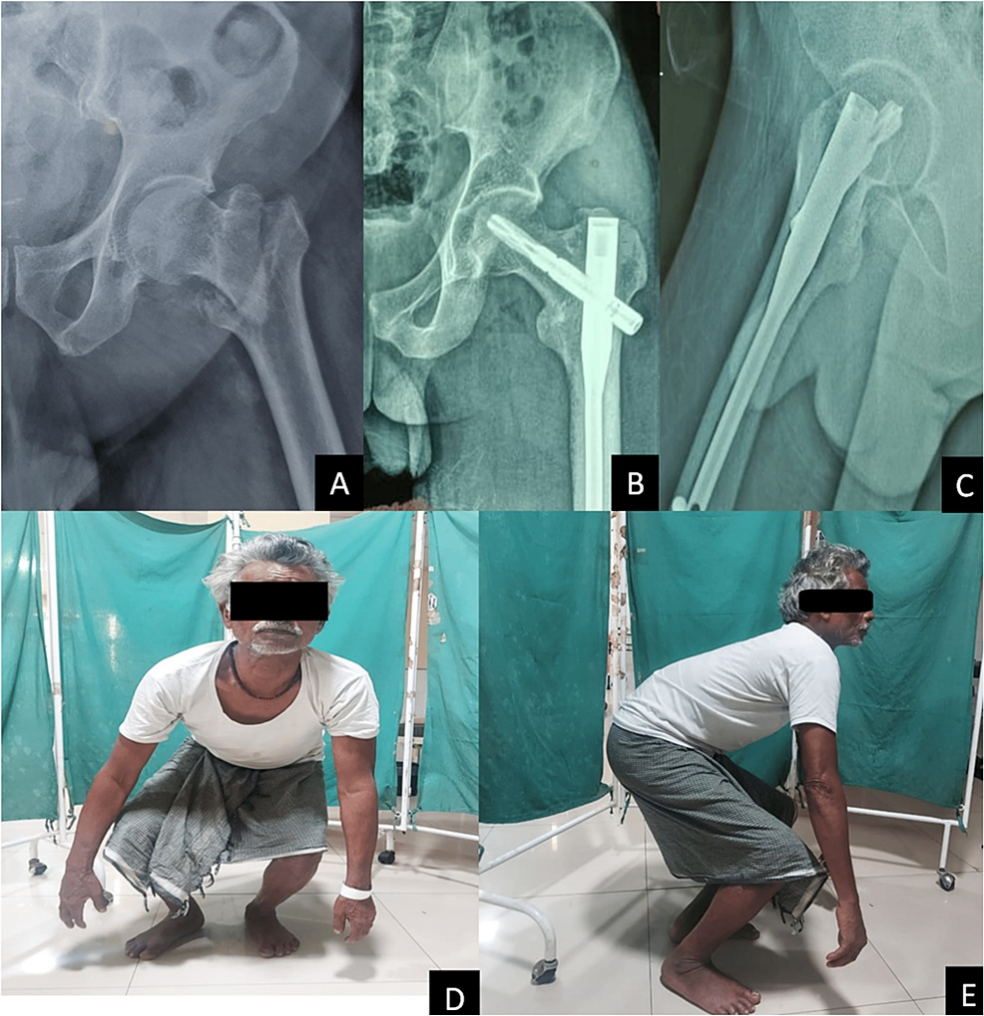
Preoperative anteroposterior (AP) view showing an intertrochanteric fracture (A), follow-up radiographs at 3 months postoperatively showing satisfactory fracture healing (B, C), and squatting without any discomfort (D, E).
